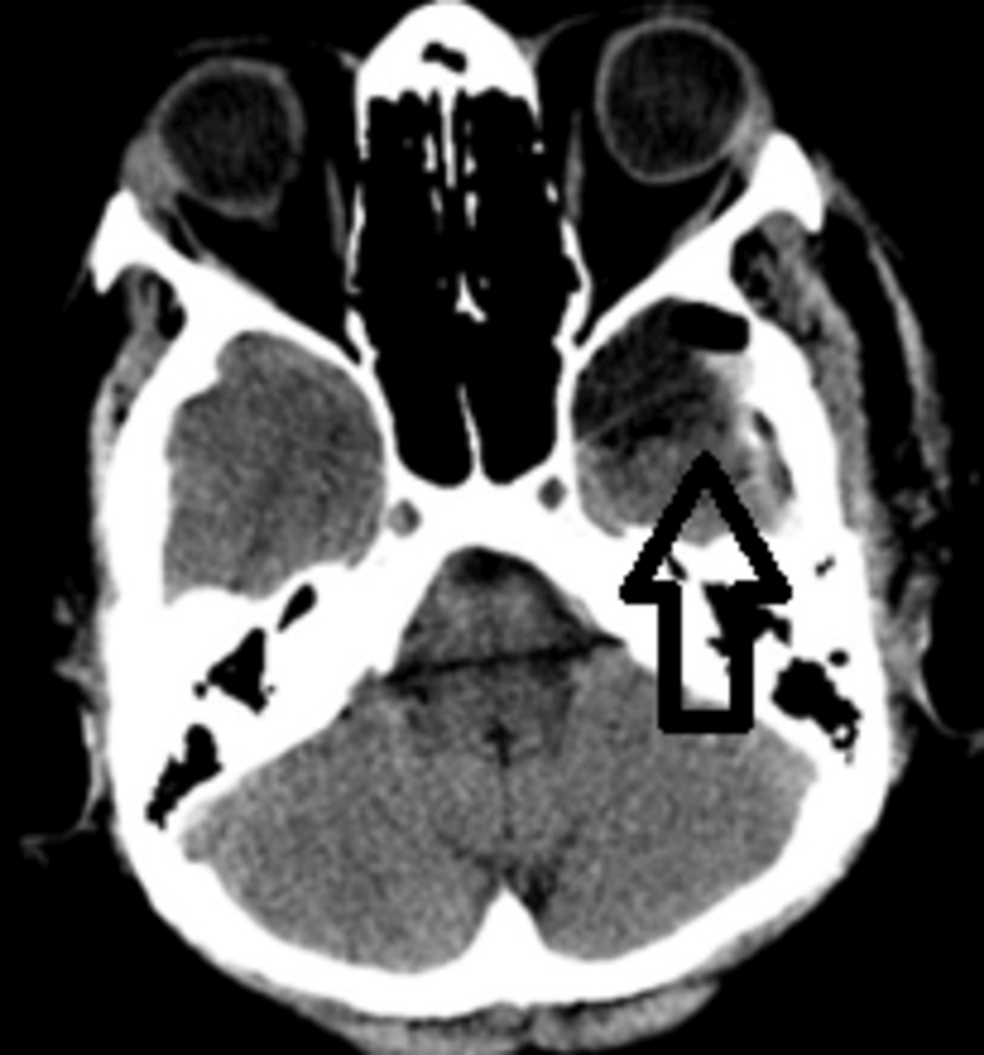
An MRI shows total extirpation of the malignant mass MRI: axial plane
